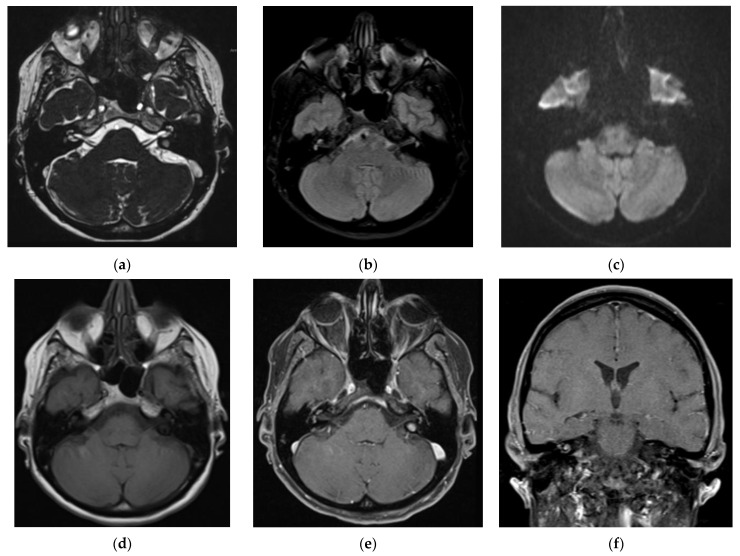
Magnetic resonance images. Hyperintense signal of cochlea, vestibule, and semicircular canals on both sides, more prominent on the right side, in axial T2W SPACE (a), coronal FLAIR (b), and axial (e) and coronal postcontrast T1WI (f), without alteration of signal intensity on axial DWI (c) and axial precontrast T1WI (d).
